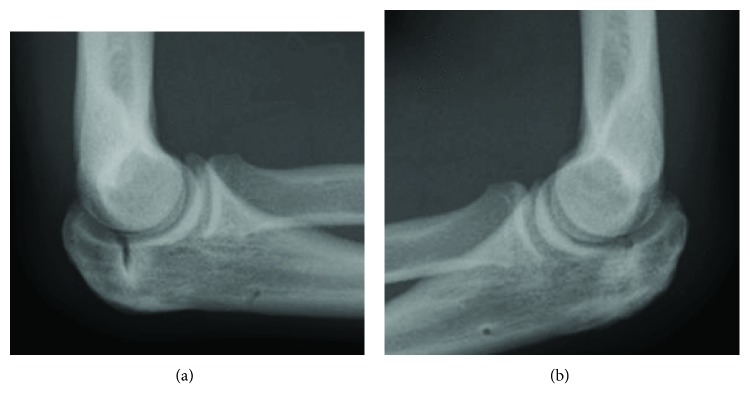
The radiographs of the elbows obtained one year after surgery. (a) Right elbow. (b) Left elbow.
